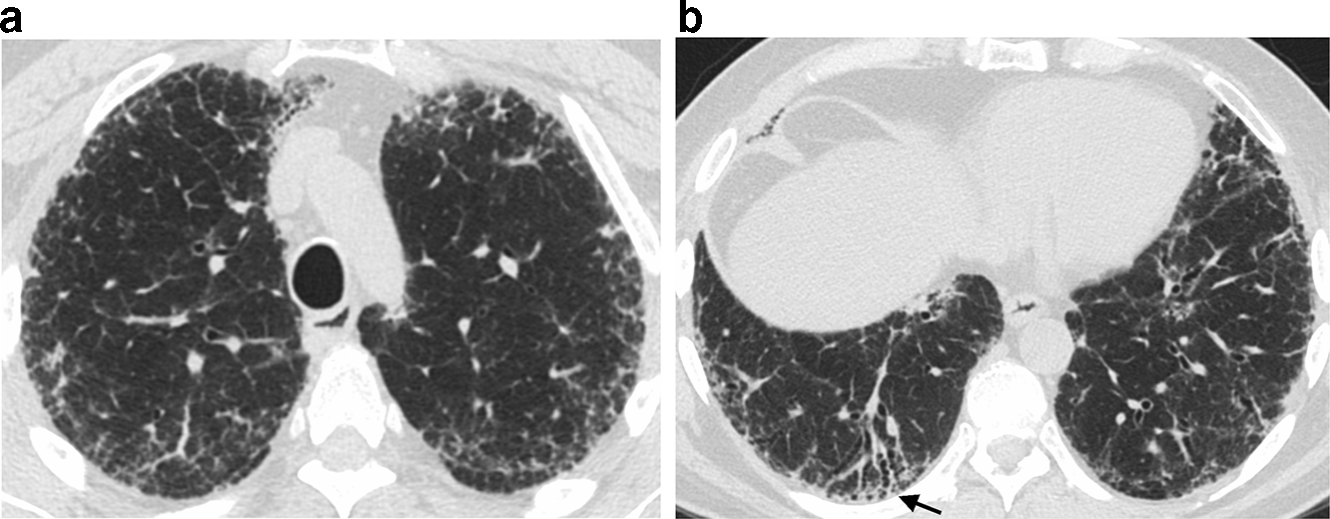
Probable UIP on HRCT. Subpleural reticular upper abnormality in the upper lungs (a) with increasing fibrosis and traction bronchiectasis (arrow) at a more caudal (b) level. The pattern appears similar to typical UIP, but lacks honeycombing, and thus is labelled probable UIP. HRCT, high-resolution CT; UIP, usual interstitial pneumonia.
